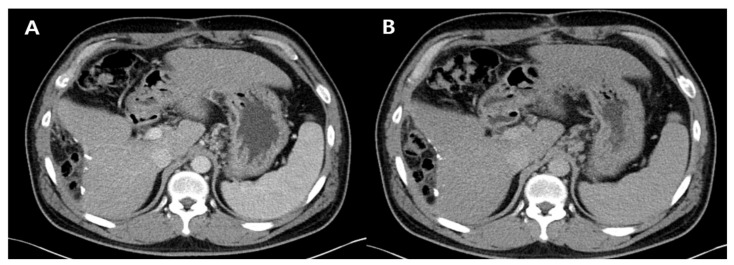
Follow-up dynamic computed tomography scan after 1 year. Multiple surgical clips at the previously resected margin are seen without new lesions: arterial phase (A) and delayed phase (B).
